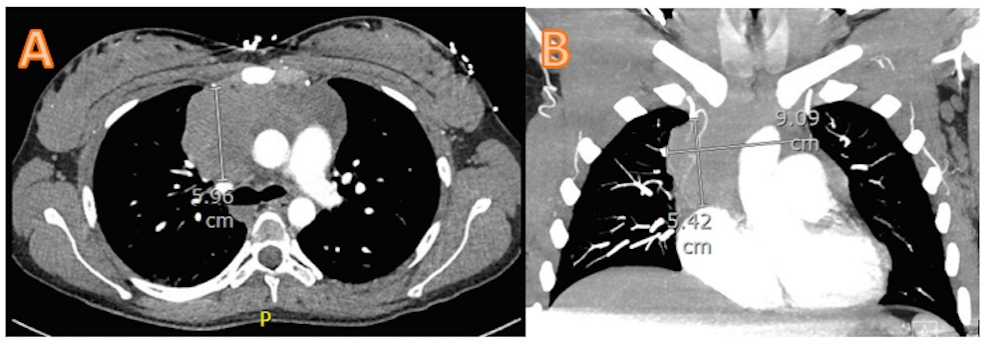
CT chest showing the decreased size of the anterior mediastinal mass: 5.96 cm in sagittal section (A) and 5.42 x 9.09 cm in coronal section (B)
